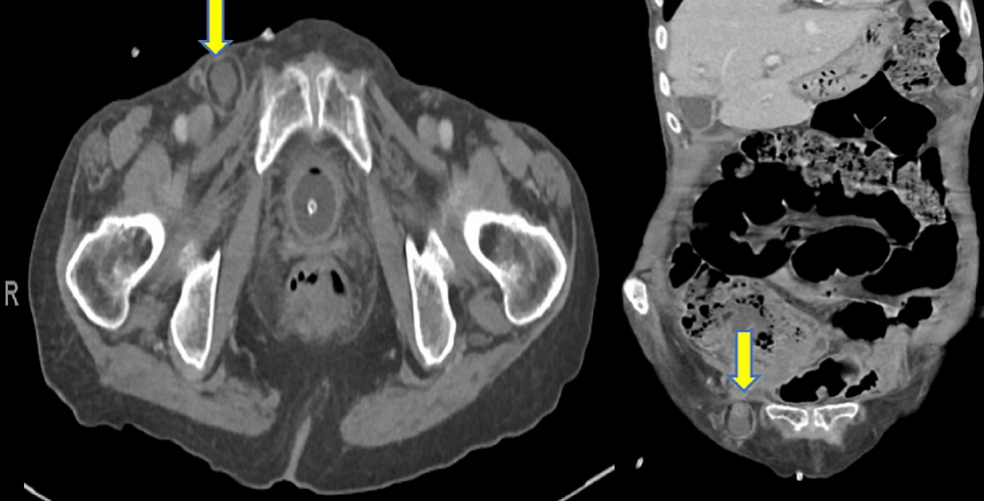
Computed tomography indicating right femoral hernial sac located inferior and lateral to pubic tubercle containing a small bowel loop with enhanced wall and free fluid (yellow arrows)
